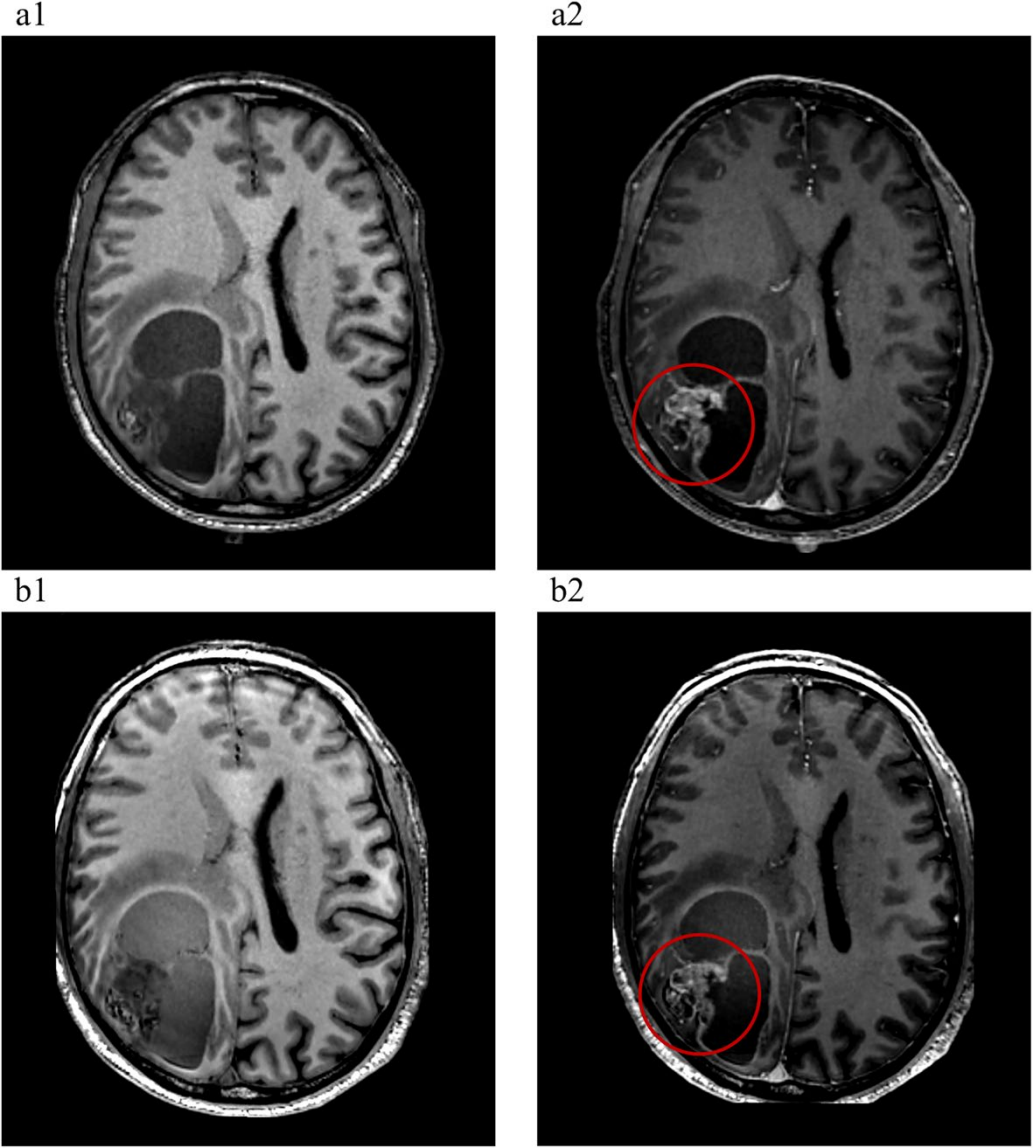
Brain metastases (lung origin), 64-year-old male. (a1) T1w MRI without contrast enhancement at 3.0 T and (b1) T1w MRI without contrast enhancement at 5.0 T. (a2) Full-dose enhanced T1w MRI at 3.0 T and (b2) half-dose enhanced T1w MRI at 5.0 T. As depicted within the red circle, the augmentation of the lesion parenchyma exhibits a slightly greater intensity at 3.0 T compared to 5.0 T. However, in terms of delineating the intricacies of the lesion, 5.0 T surpasses 3.0 T due to its employment of a thinner scanning layer thickness and higher resolution
